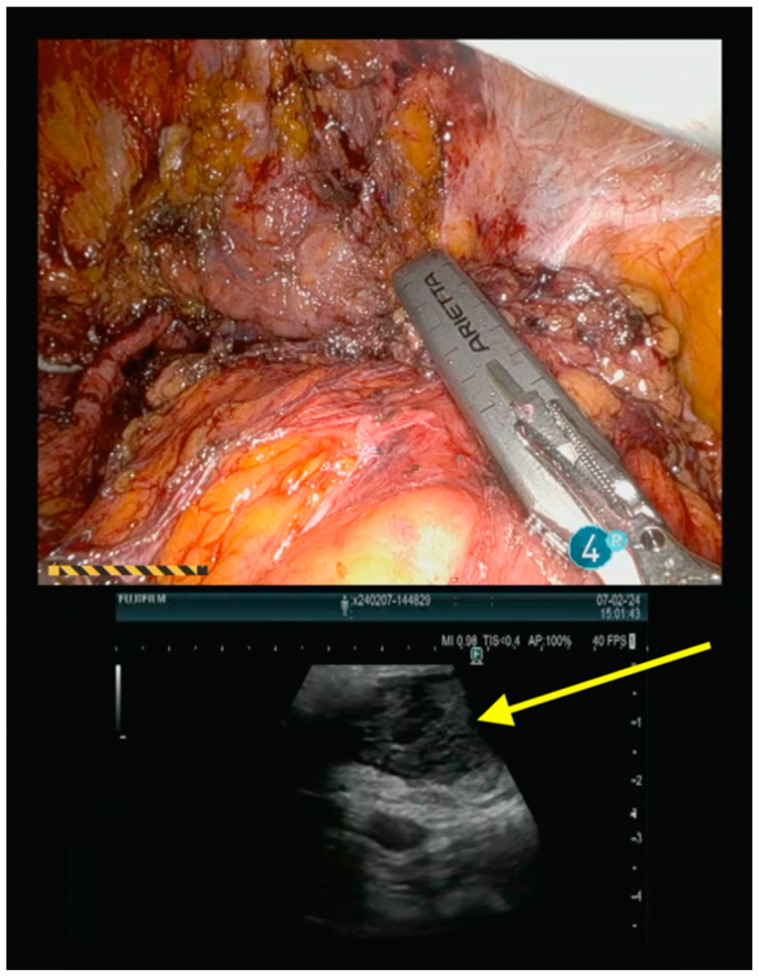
Intraoperative ultrasound, showing a 4 cm vascularized lesion of the sigma rectum. The precise localization of the recurrence allows for complete disease resection with clear surgical margins.
